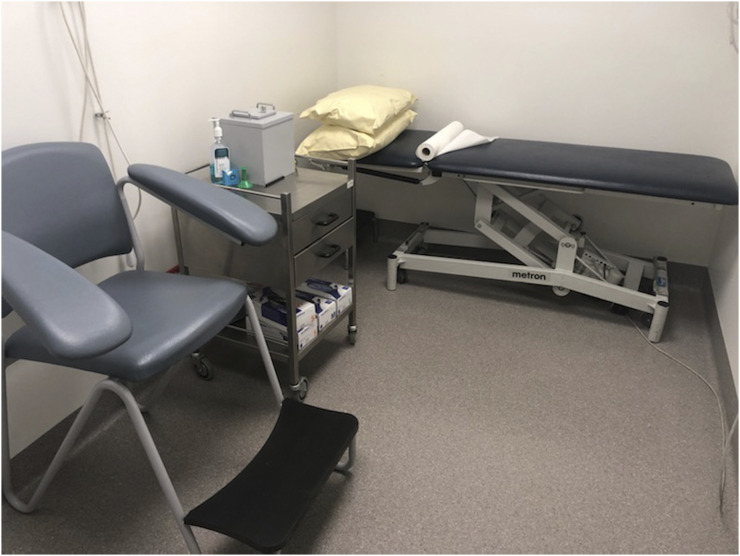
New normal for nuclear medicine injection room, with absence of linen (replaced by single-use paper roll), decluttered environment, and readily sanitized sealed surfaces. (Courtesy of Queensland Xray.)
